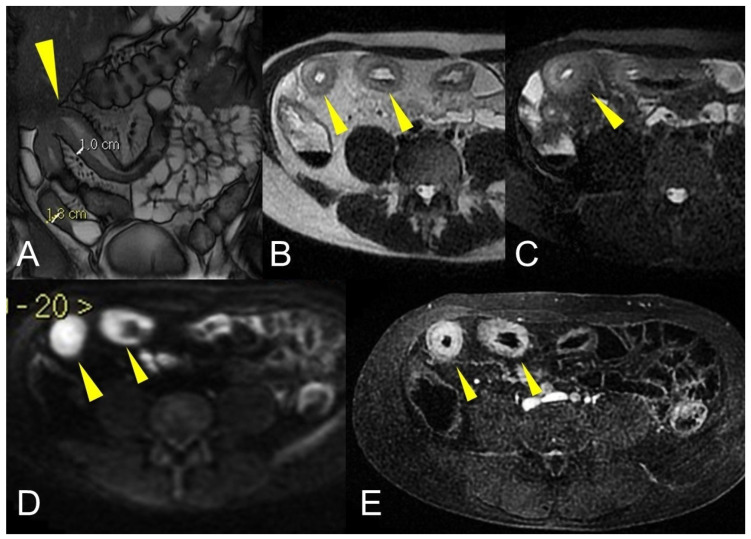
Active inflammatory Crohn’s disease component revealed by MRE imaging. Coronal fast imaging employing steady-state acquisition (FIESTA) image (A) show mural thickening involving the terminal ileum (yellow arrowhead). Axial T2-weighted MRE image (B) of the same patient shows small bowel hyperintense wall thickening (yellow arrowheads) with matching parietal edema, restricted diffusion, and homogeneous enhancement, respectively in axial T2-weighted images with fat suppression ((C), yellow arrowheads), axial diffusion-weighted image ((D), yellow arrowheads), and contrast-enhanced fat-suppressed T1-weighted image ((E), yellow arrowheads).
